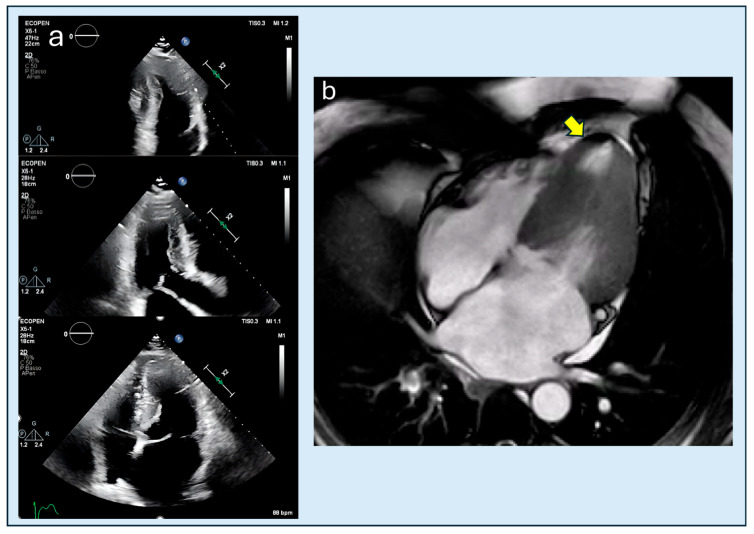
54-year-old male patient with HCM. (a) TTE failed to detect the apical aneurysm, likely due to limitations related to suboptimal acoustic windows and reverberation artifact. (b) CMRi clearly demonstrates the early aneurysmal remodeling of the apical septum (yellow arrow). HCM, hypertrophic cardiomyopathy; TTE, transthoracic echocardiography; CMRi, cardiac magnetic resonance imaging.
